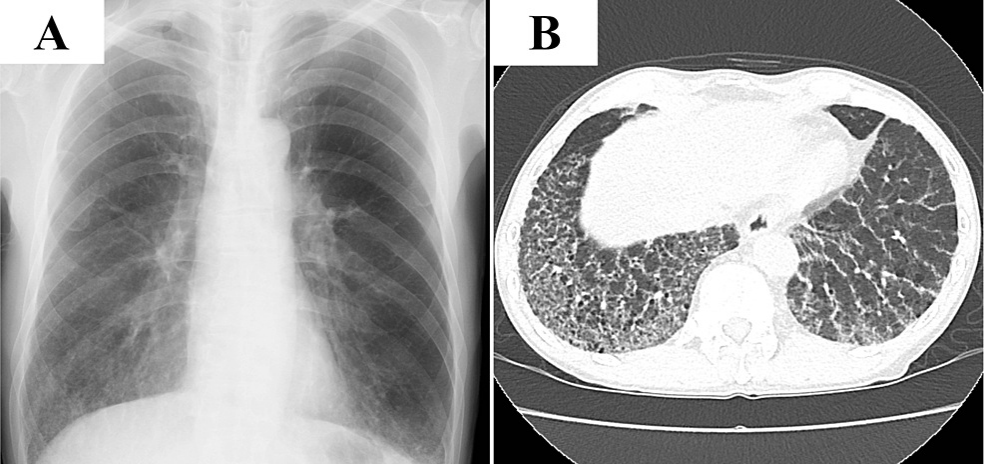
Chest X-ray and chest high-resolution computed tomography at initial examination. A: Chest X-ray showed ground-glass opacity in the lower lung field. B: Chest high-resolution computed tomography: reticular and ground-gross shadows extend along the bronchovascular bundles and just below the pleura in the lower lobes of both lungs.
